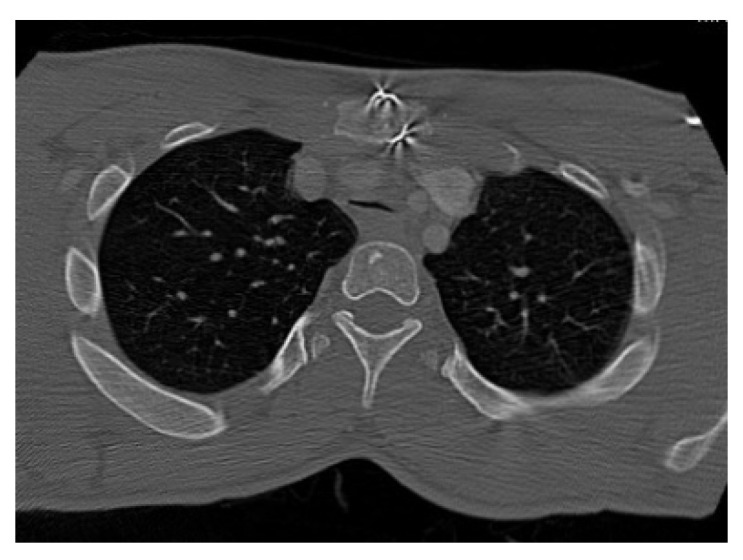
CT scan of MPS II showing collapsed trachea, suggestive of tracheomalacia.
